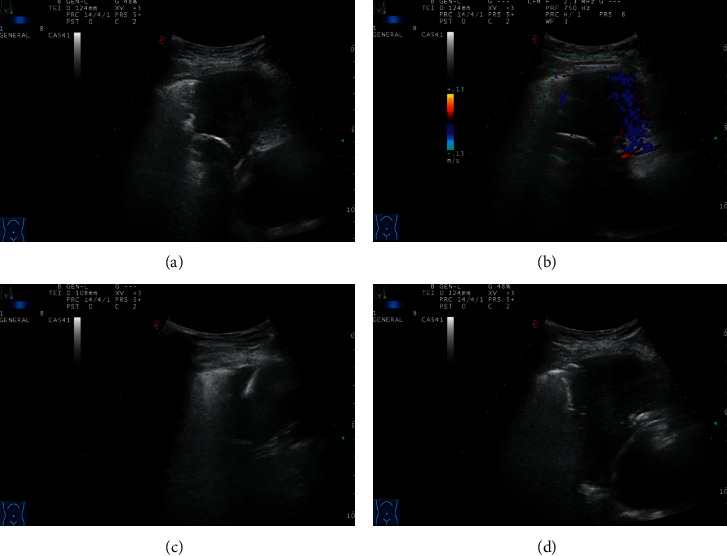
A patient in the conventional ultrasound group, male, 56 years old. (a) Ultrasonography showed a hypoechoic mass in the middle lobe of the right lung, with a size of 51 mm∗38 mm, and the echo was still uniform. (b) Color Doppler displayed a little blood flow signal. (c) The needle was passed through the pleura, into the lesion, and cut for biopsy. (d) Five minutes after needle extraction, no obvious bleeding and pneumothorax and other complications were found.
